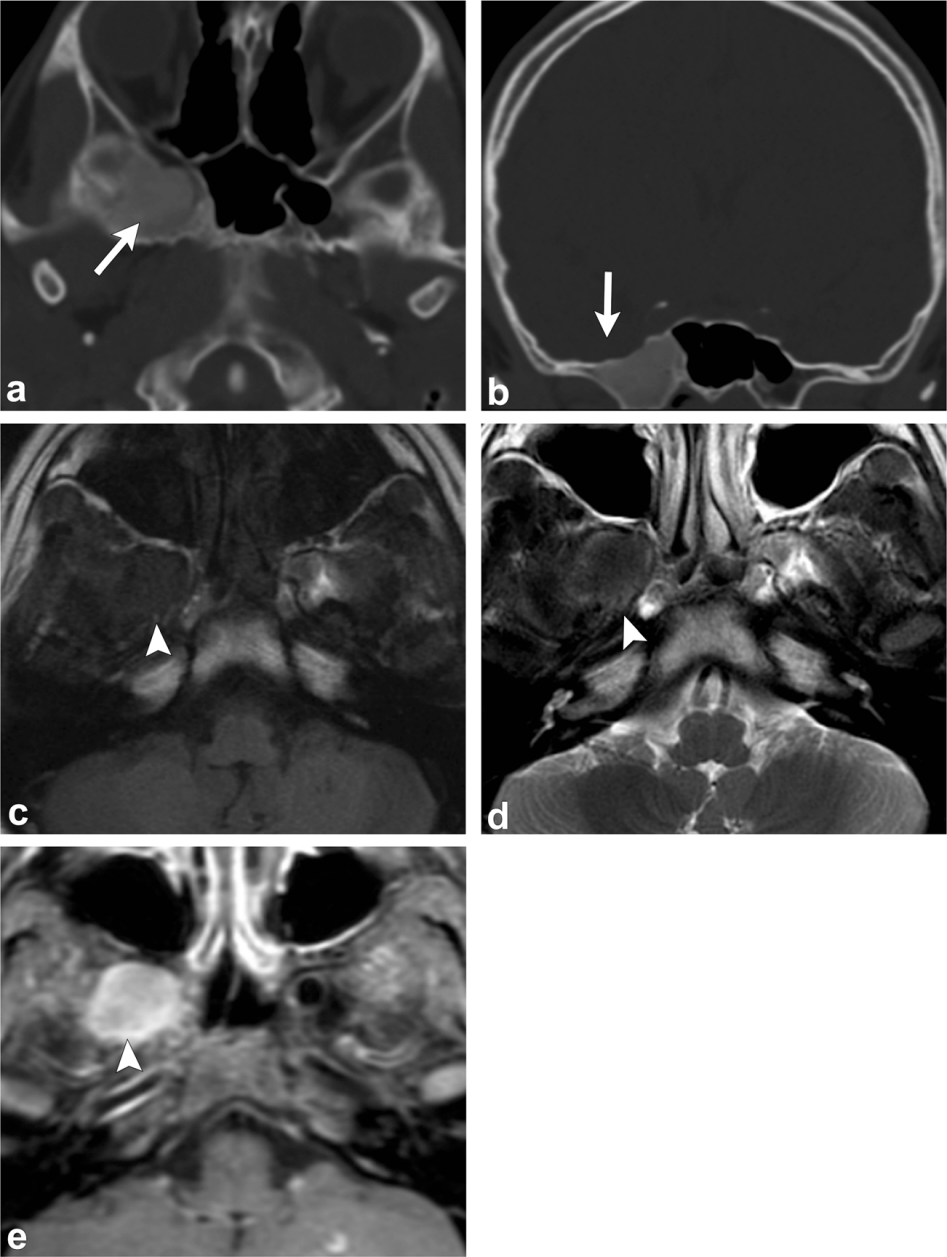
Fibrous dysplasia. Axial (a) and coronal (b) head CT images demonstrate an expansile lesion with ground-glass matrix in the right greater wing of the sphenoid (*arrows*). Axial T1-weighted (c), axial T2-weighted (d) and axial post-contrast T1-weighted (e) images show that the lesion is hypointense with homogeneous enhancement (*arrowheads*)
